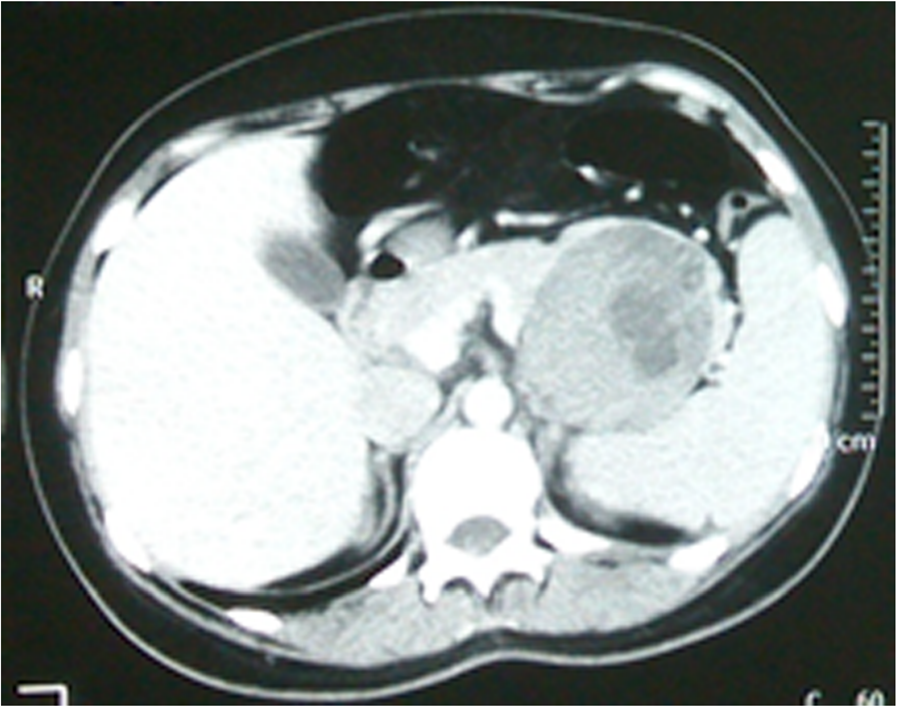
Abdominal CT with IV contrast showing neoplasm of the pancreatic tail in close contact with the spleen.
